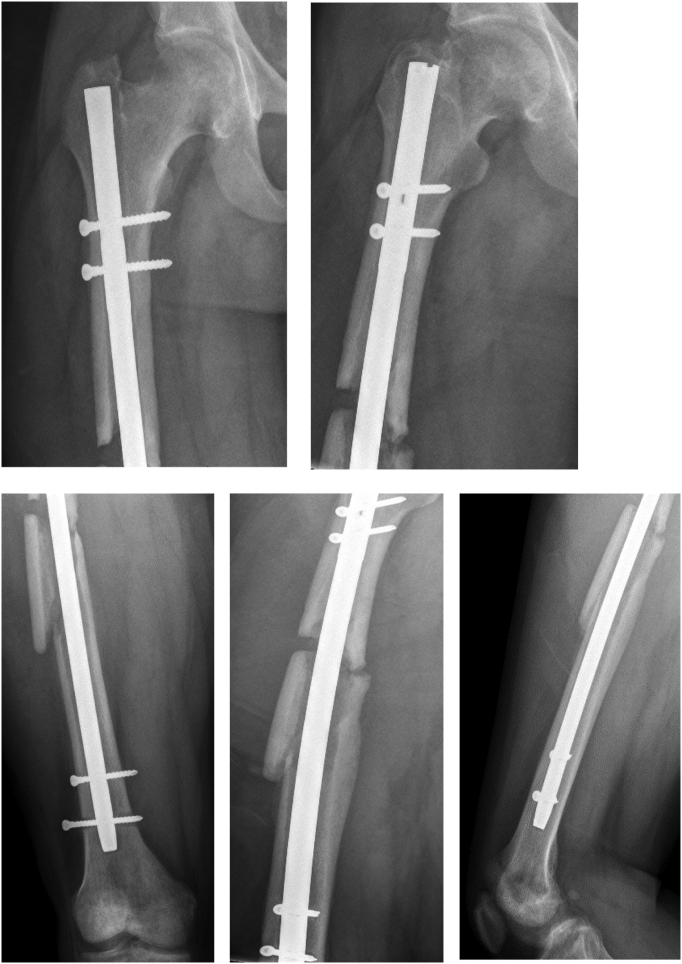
Patient's radiographs upon initial presentation for his infection in the US with original IM nail from Uganda in place.
